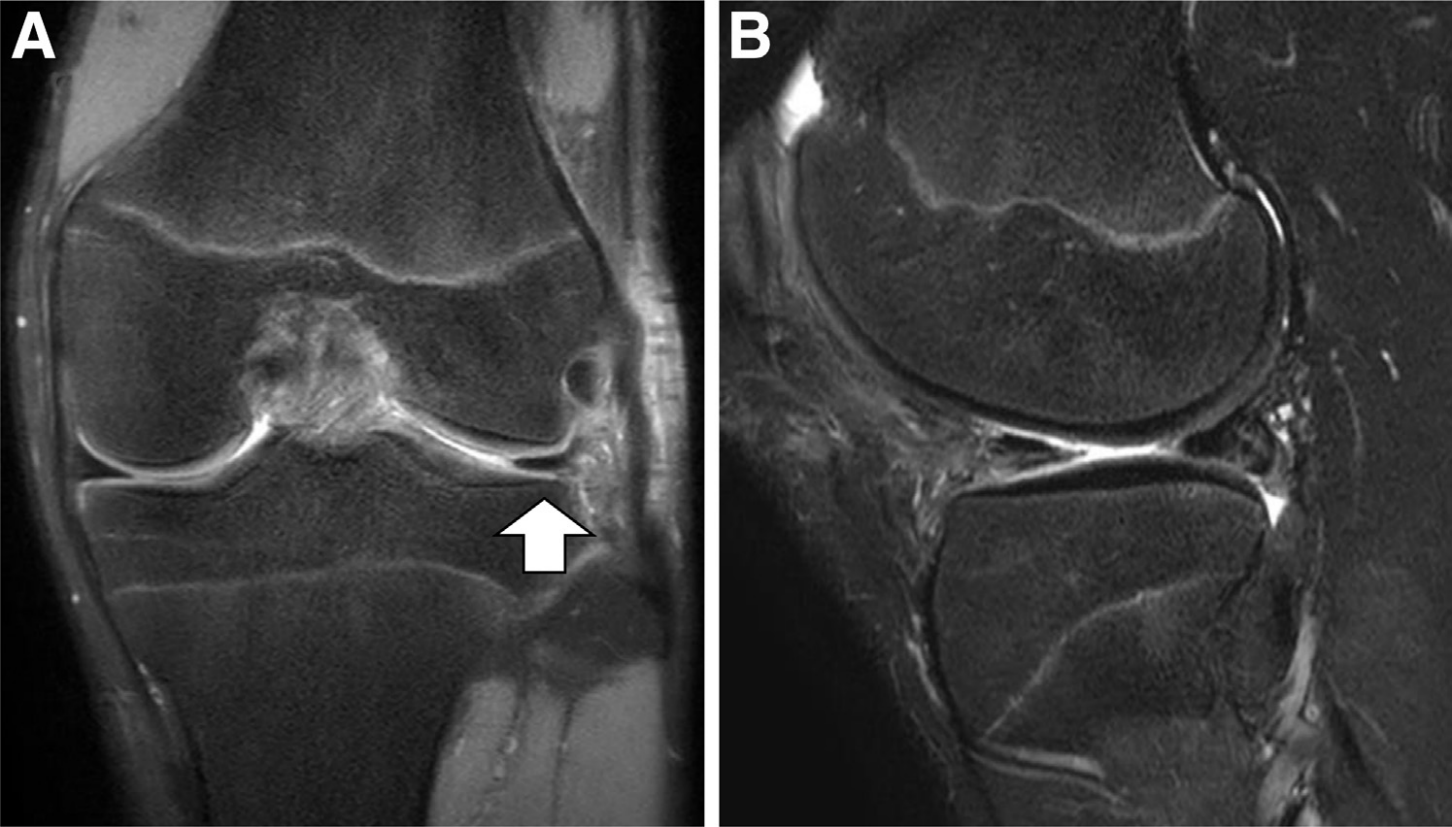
Postoperative magnetic resonance imaging after saucerization with the transportal transcapsular repair for anterior instability and additional inside-out suture for consecutive posterior instability in the left knee. It resembled the normal meniscus. The width of the body of the lateral meniscus was 10 mm (A; white arrow).
